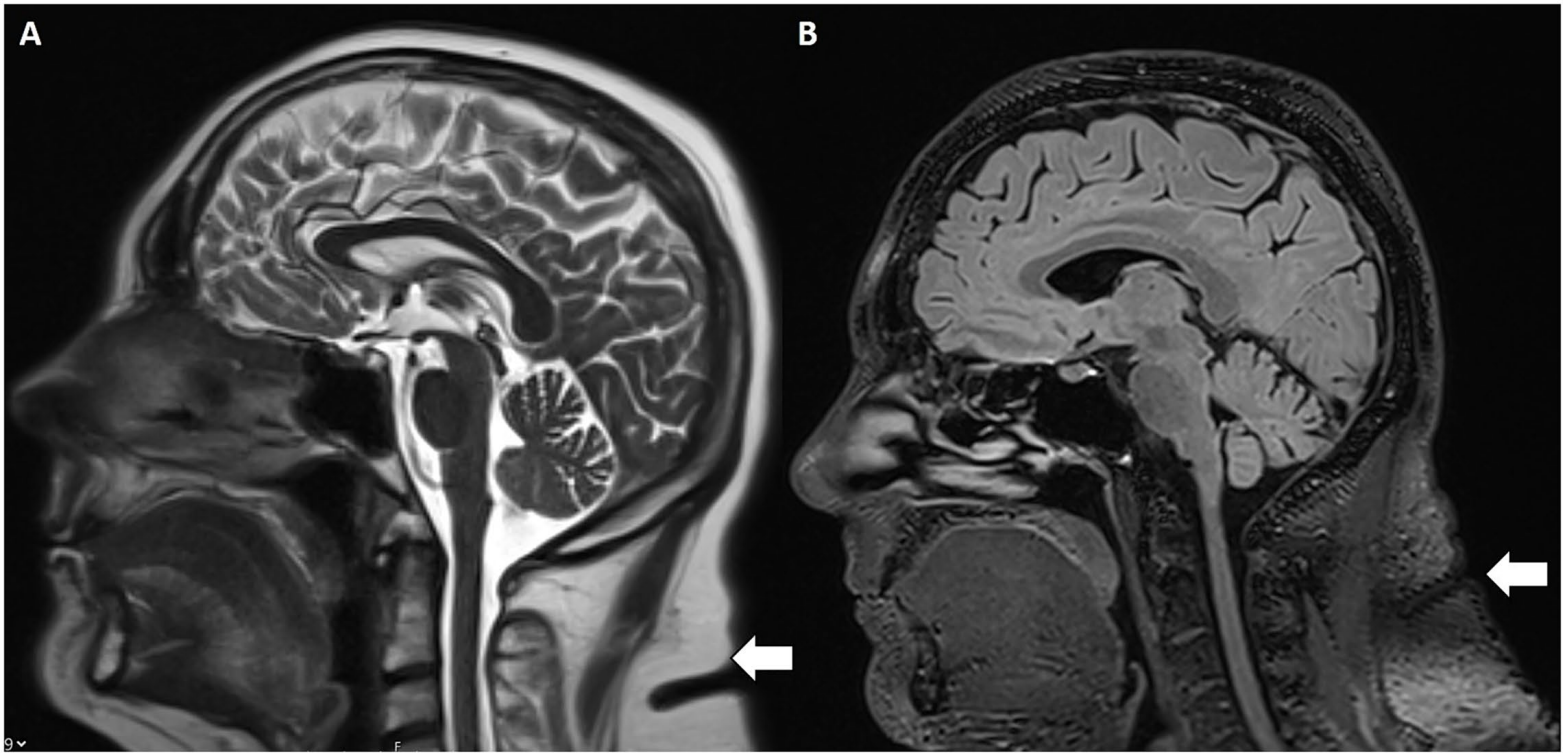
Increased subcutaneous nuchal fat. Sagittal T2w (A) and FLAIR (B) MRI with increased subcutaneous fat (white arrow) at the C2–C3 intervertebral disc space.
